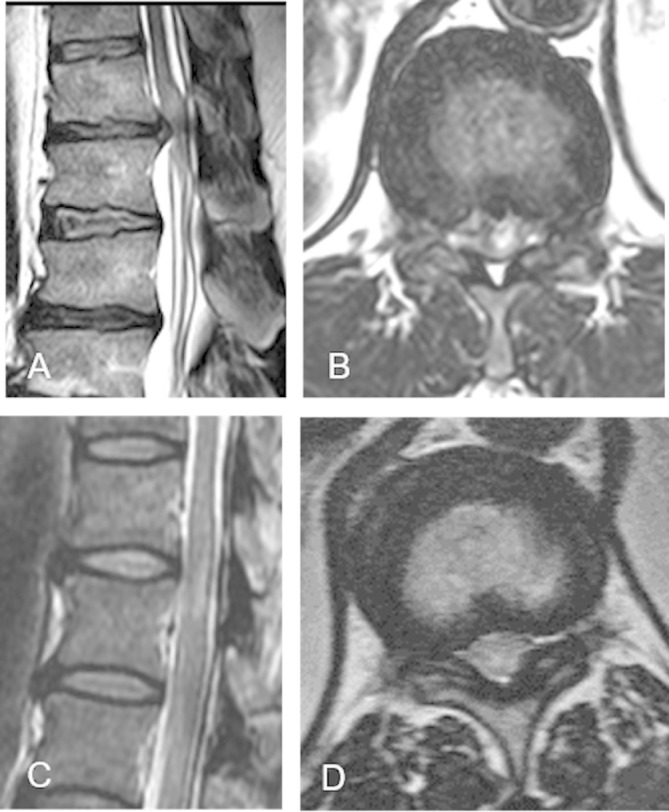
Sagittal T2-weighted MR scan of spine of case 1 (A) and case 2 (C), showing intramedullary signal hyperintensity at T11/12 in case 2 (C). Corresponding axial T2-weighted MRI showing a disc protrusion at T11/T12 level in case 1 (in association with intramedullary signal change) (B) and ligamentum hypertrophy at T11/T12 level in case 2 (D).
